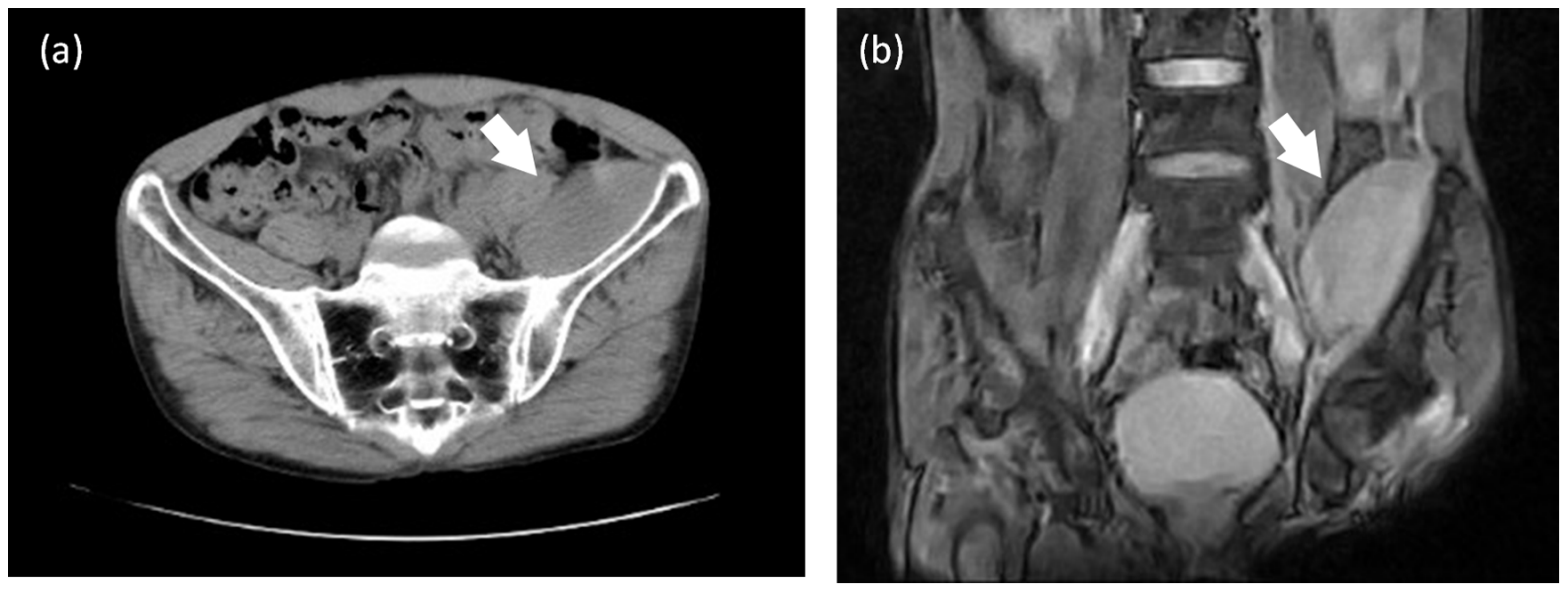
Pelvic computed tomography of the RA patient with factor XIII deficiency. The white arrow indicates the intra-pelvic hemorrhage. (a) Transverse plane and (b) coronal plane.
